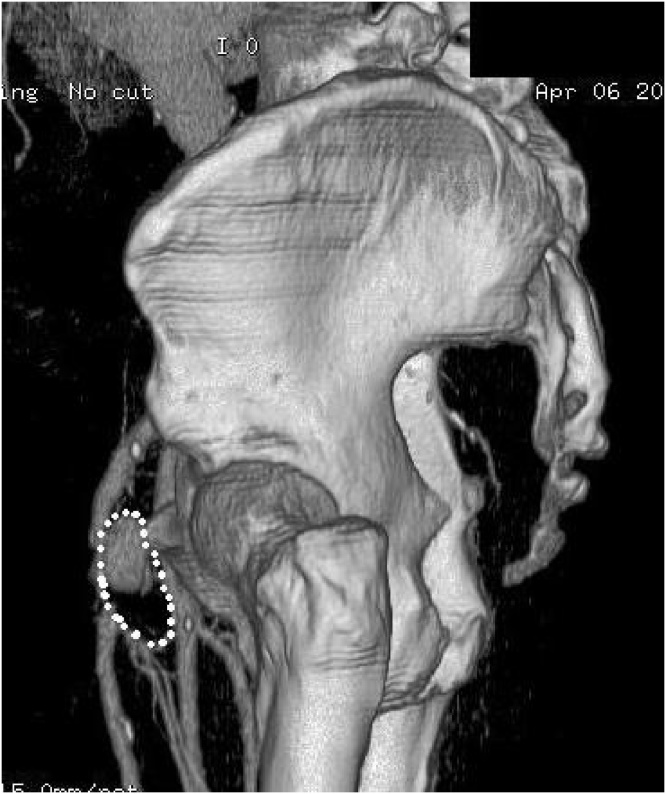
A 3-dimensional reconstruction of the right femoral aneurysm in this patient as shown on CT angiogram. The actual size of the aneurysm including thrombus outlined.
